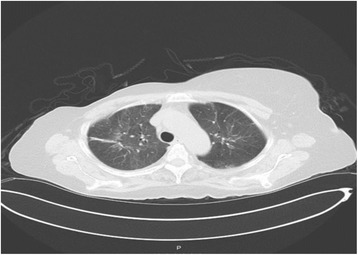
Patient’s chest computed tomography after stopping methotrexate and starting steroids
